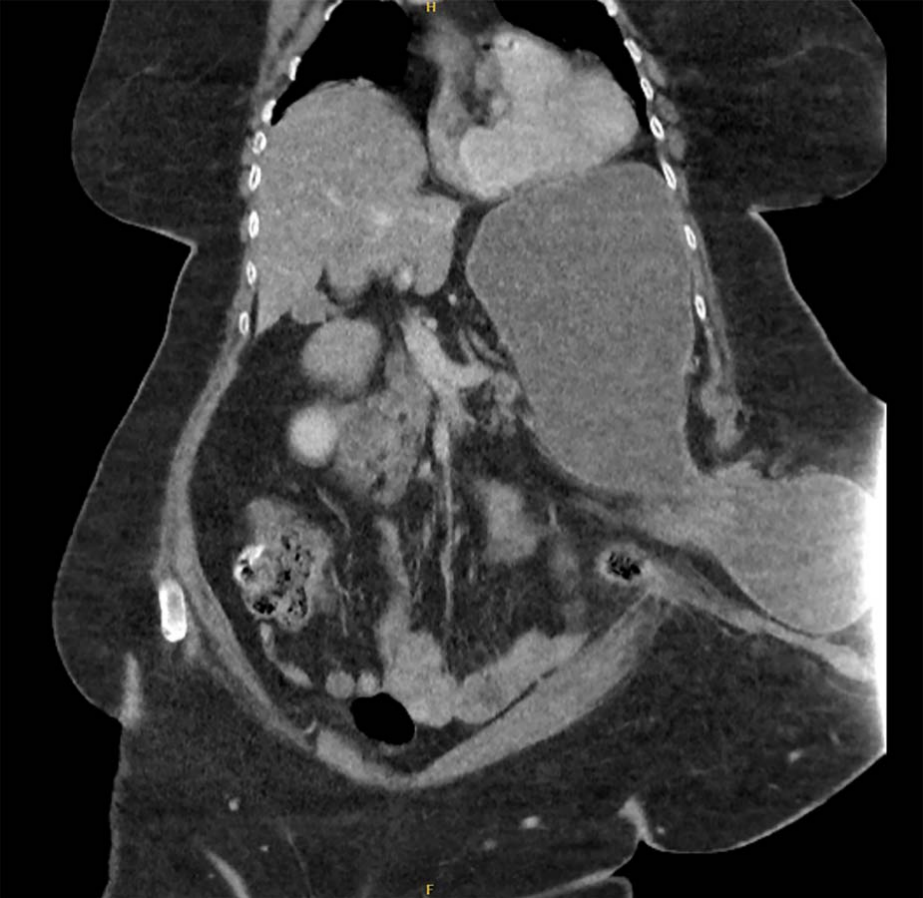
CT abdomen pelvis—coronal view of parastomal hernia containing stomach.
